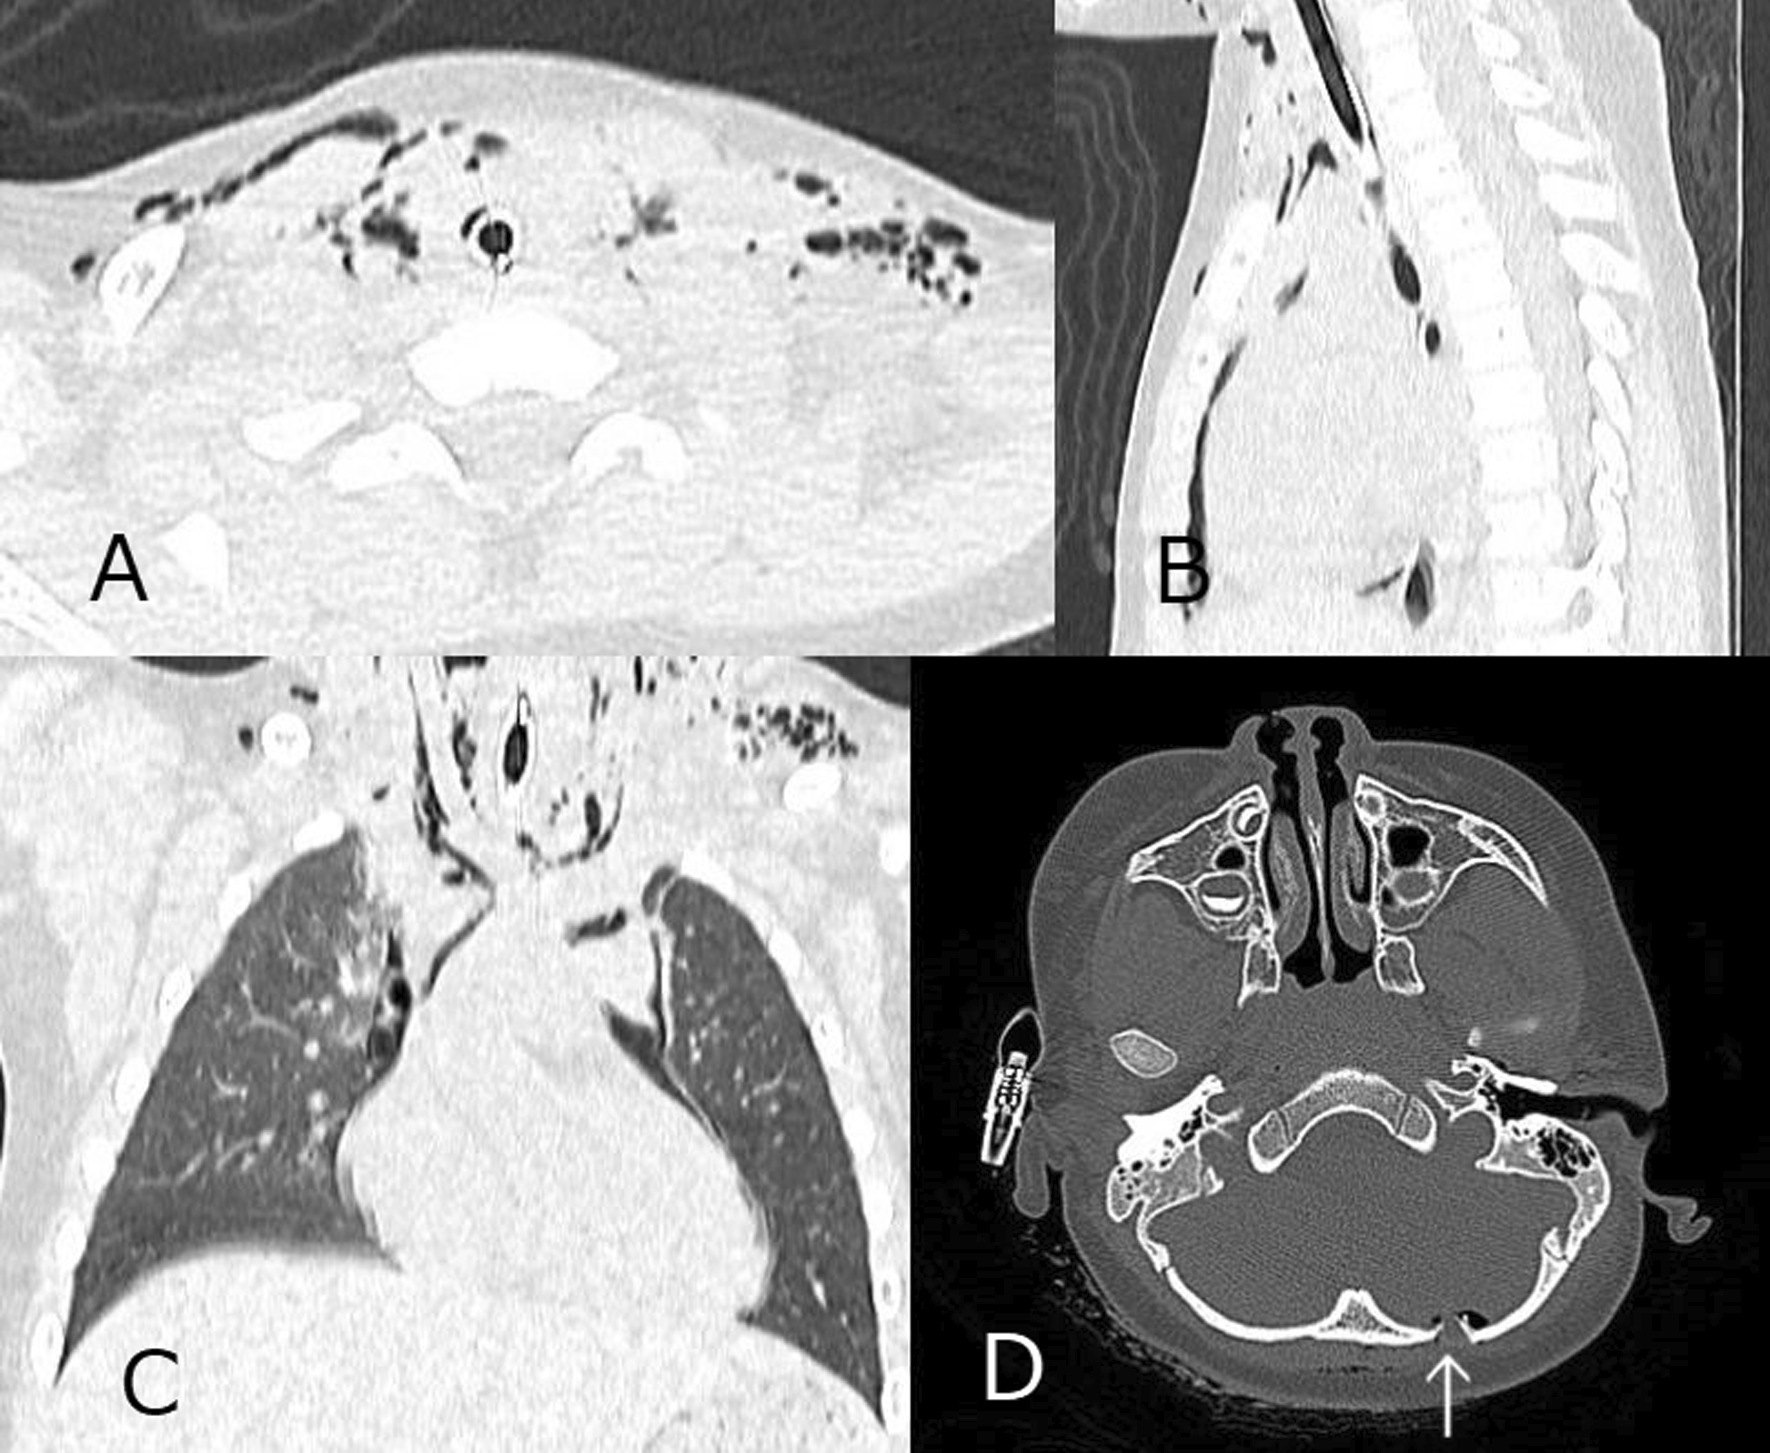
A, B, C Computed tomographic scan of the chest shows subcutaneous and mediastinal emphysema, but the area of tracheal injury is not clear as the tube has already been implanted. DComputed tomographic scan of the head shows a depressed skull fracture and a small amount of air in the intracranial space (arrow)
